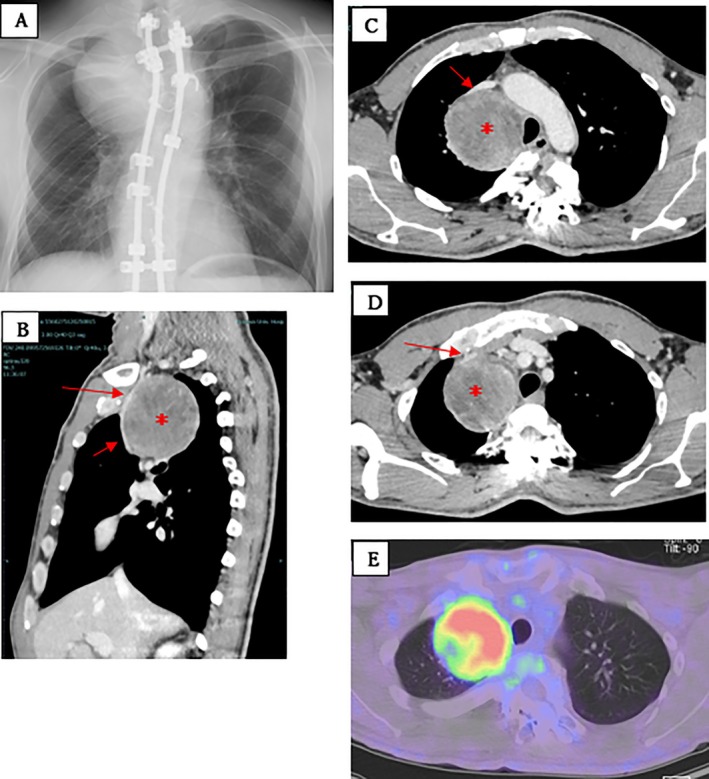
Imaging findings. Chest radiography showed a mass in the right upper to middle lung fields with tracheal deviation (A). CT demonstrated a mass (asterisk) in the right middle mediastinum compressing the SVC (short arrow) and right brachiocephalic vein (long arrow) (B–D). PET–CT showed FDG uptake (SUVmax, 8.5) (E).
